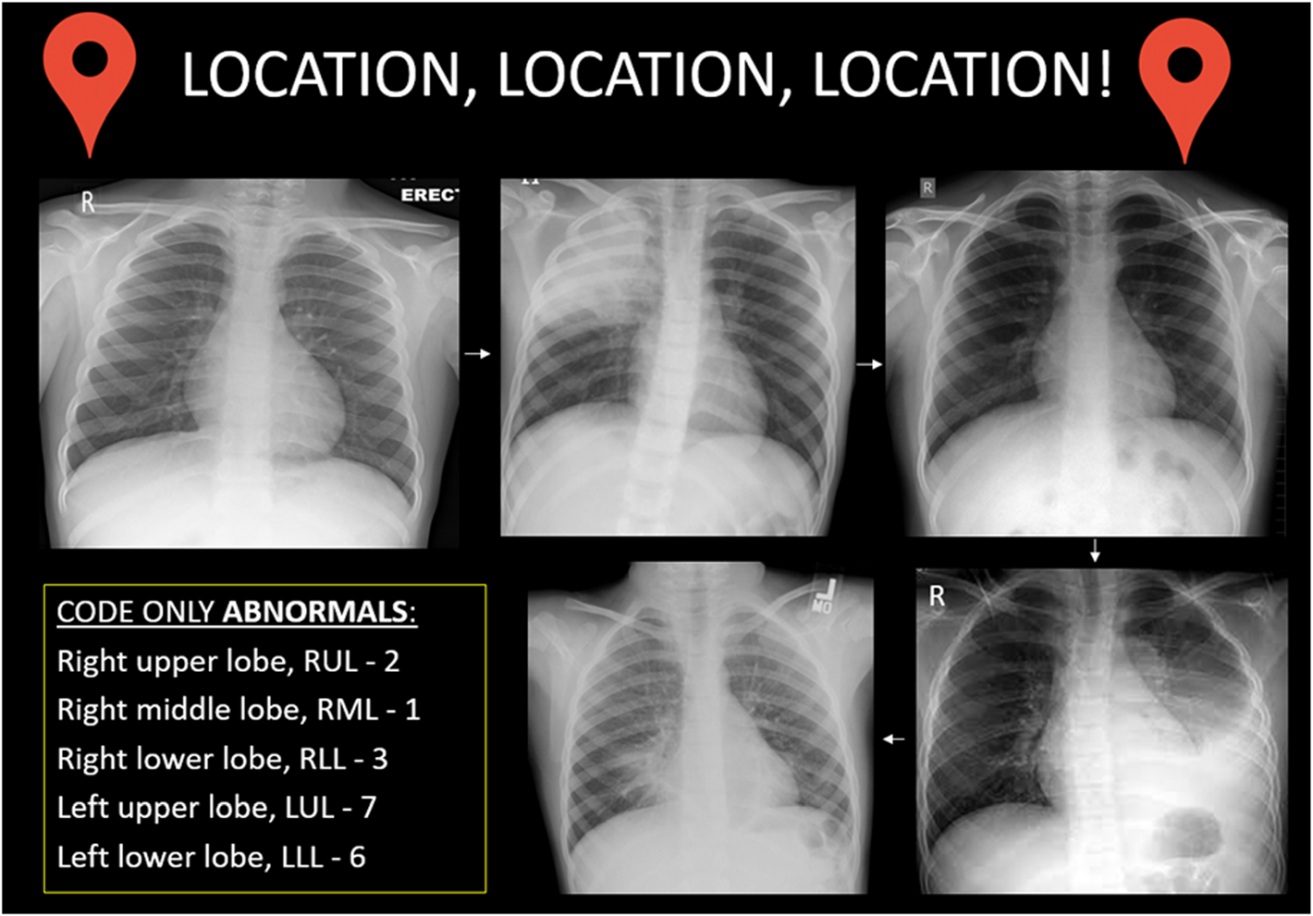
‘Location, location, location’: another example of a puzzle in the escape room. The participants need to identify whether a chest radiograph is normal or abnormal. If abnormal, then the location of the consolidation should be determined and matched to the number code given in the box in the bottom left corner of the poster. For example, in this game, the three number combination is 2, 6 and 1
